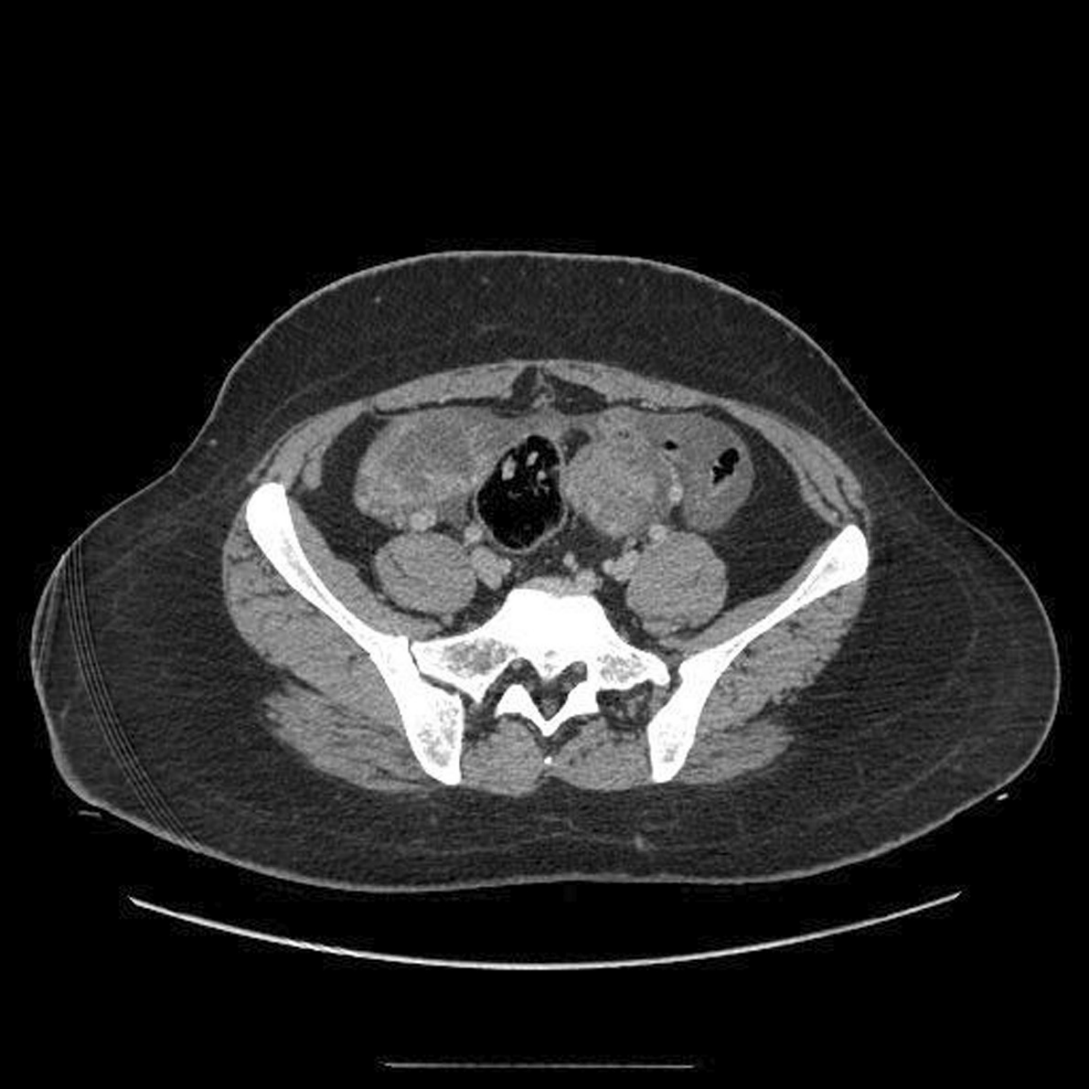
Pelvic CT scan showcasing bilateral ovarian mass
